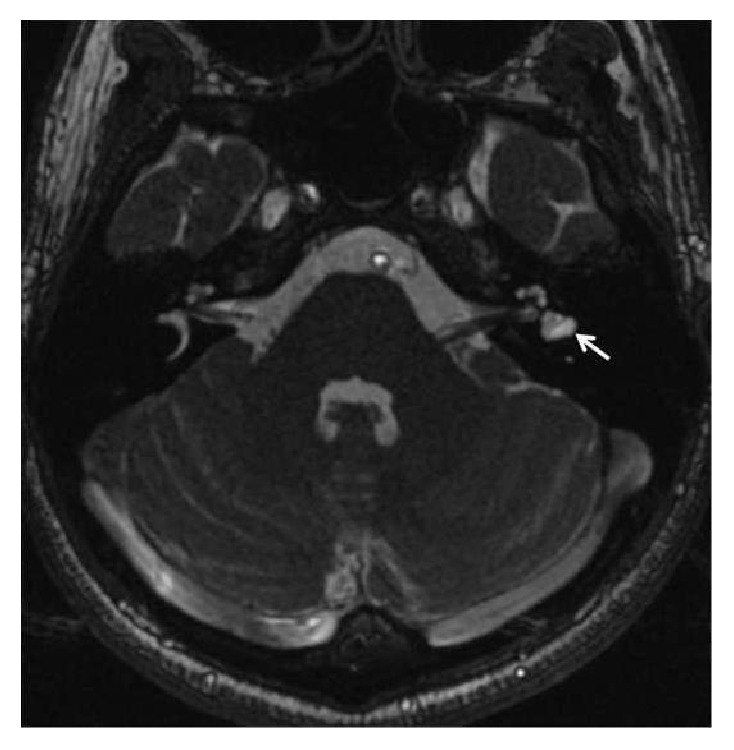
MRI of the patient showing a malformation of the left horizontal semicircular canal and vestibule forming a vestibular common cavity, compatible with the concept of lateral semicircular canal vestibule dysplasia (LCVD).
